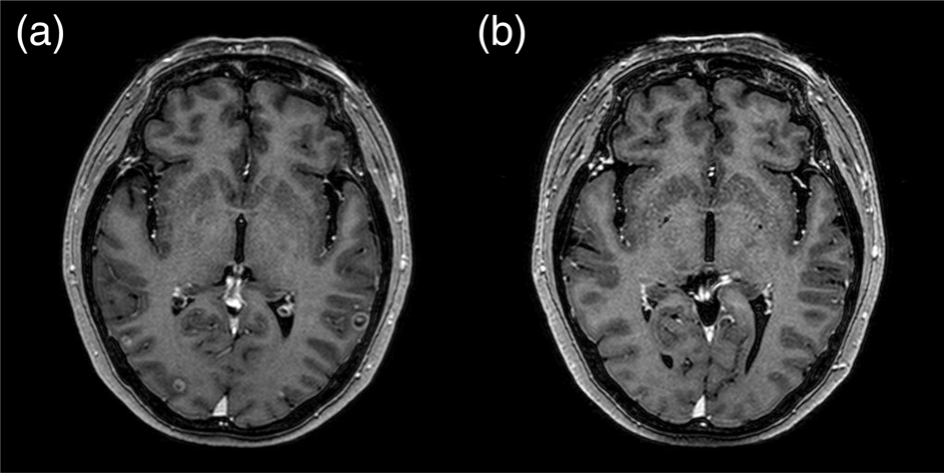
(a) Brain magnetic resonance imaging (MRI) revealed multiple brain metastases prior to treatment; and (b) the metastases almost completely disappeared following administration of osimertinib.
